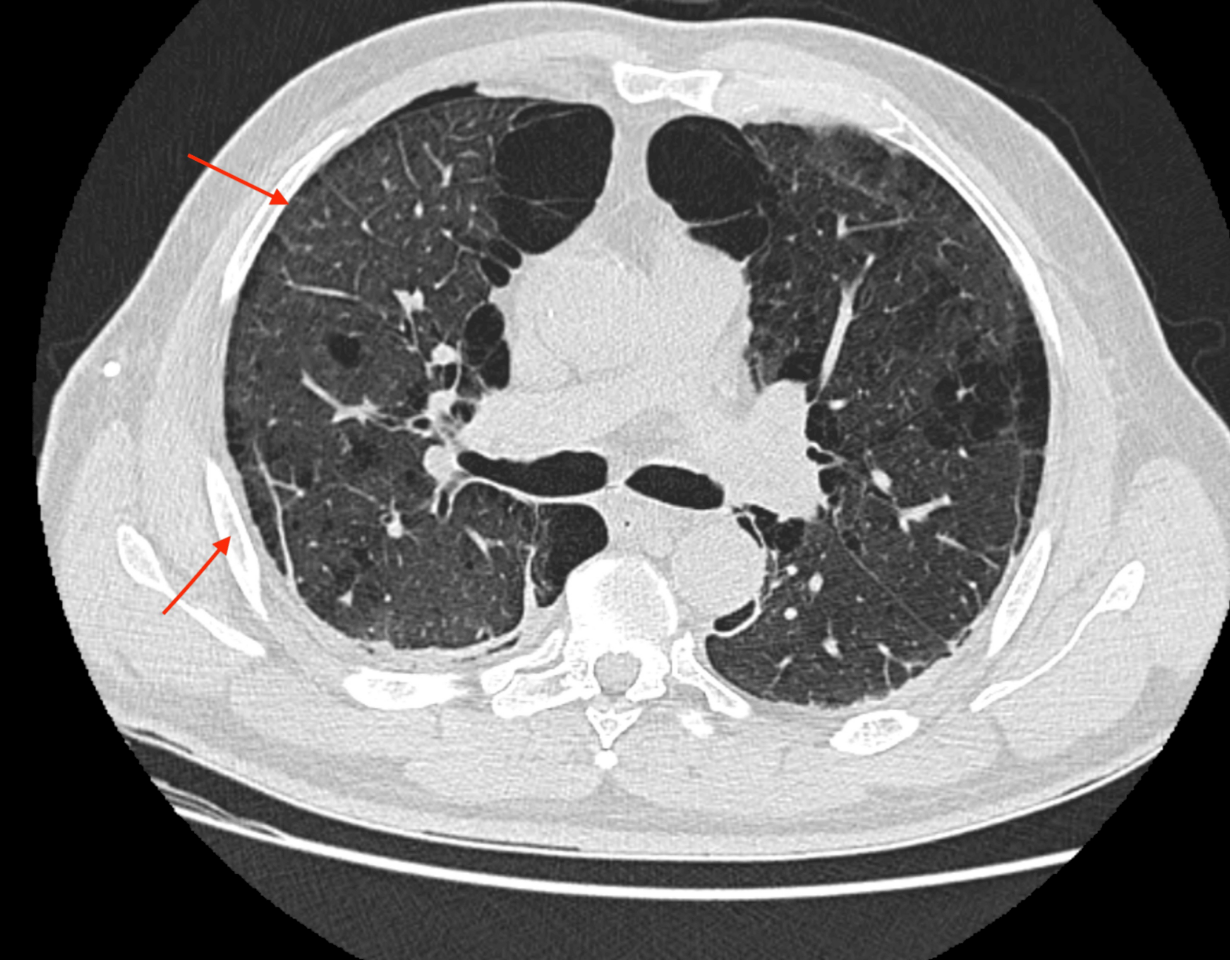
Follow-up CT confirming absence of pneumothorax and stable emphysema. CT: Computed tomography
